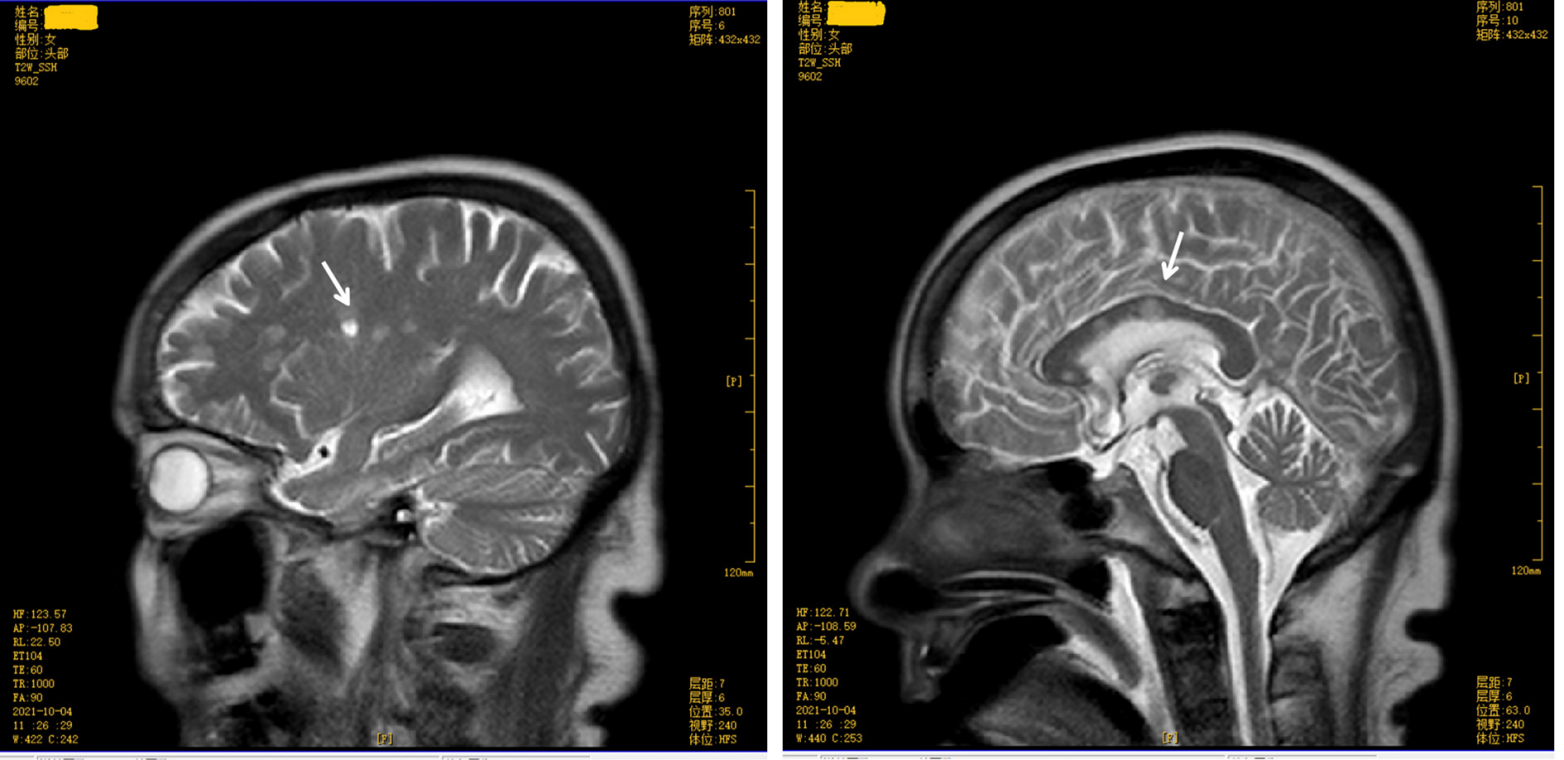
Brain magnetic resonance imaging (sagittal view) T2 weighted image shows multiple abnormal oval-shaped hyperintense signal lesions in the cerebral hemisphere and mesolobus.
